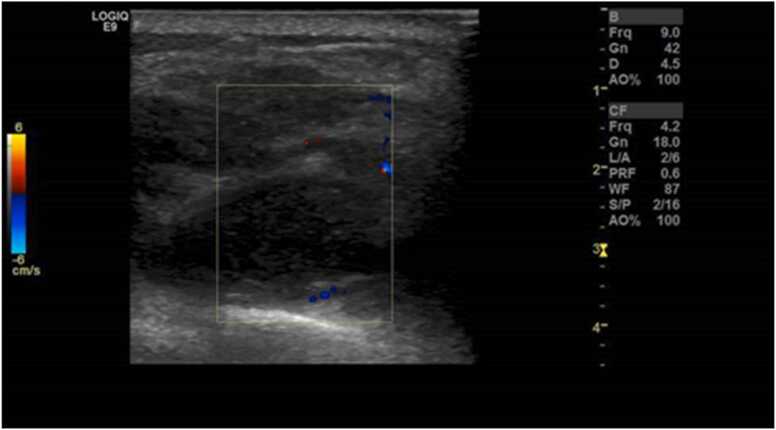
Ultrasonography showed 32 mm fluid collection over the left Acromioclavicular joint, with increased vascularity.
